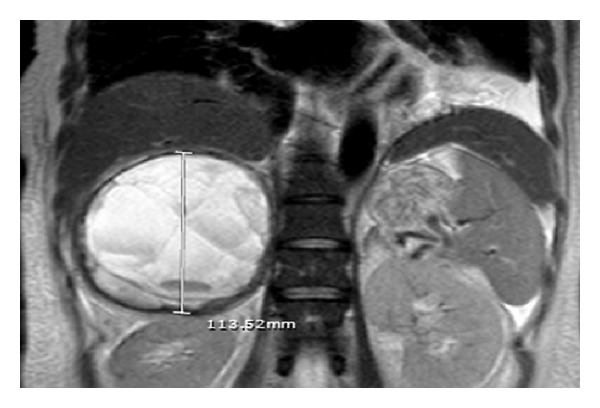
MRI abdomen (T2-weighted) of right adrenal mass.
